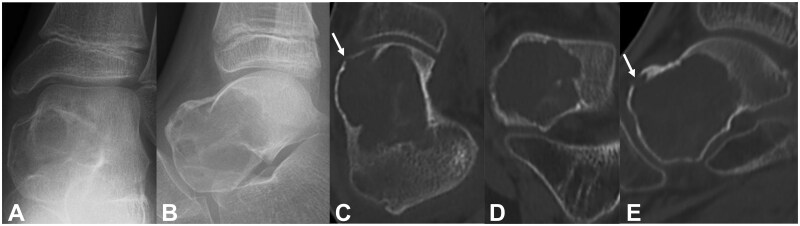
(A and B) An X-ray examination revealed a radiolucent lesion in the left talus. (C, D, and E) Bone radiolucency and cortical thinning were observed in the left talus. Findings suggestive of a pathologic fracture were observed (white arrow).
